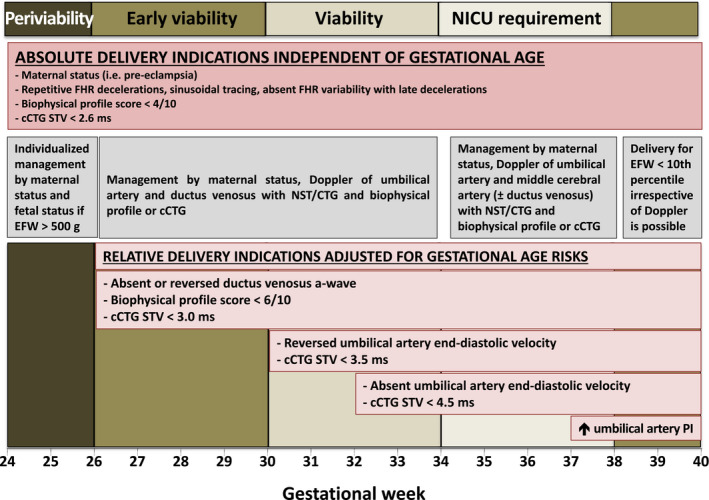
Delivery criteria for fetal growth restriction. Delivery criteria are based on monitoring with umbilical artery, ductus venosus, and middle cerebral artery Doppler at specified gestational ages with traditional nonstress testing or computerized CTG (cCTG) if available. Abbreviations: NICU, neonatal intensive care unit; FHR, fetal heart rate; CTG, cardiotocogram; STV, short‐term variation; ms, milliseconds; EFW, estimated fetal weight; PI, pulsatility index. [Colour figure can be viewed at wileyonlinelibrary.com]
